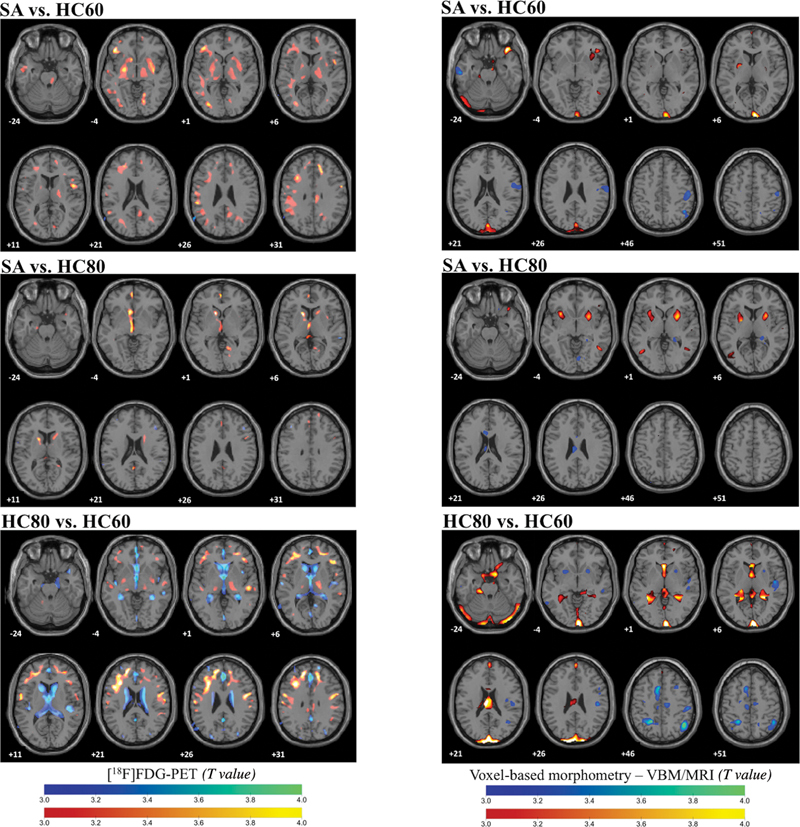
Abbreviations: GMV, gray matter volume; HC80, age-matched healthy controls; HC60, healthy controls aged 60 to 69 years; MRI, magnetic resonance imaging; rBGM, regional brain glucose metabolism; SA, SuperAgers; SPM8, Statistical Parametric Mapping 8. Note: Smaller clusters identified in the lateral ventricles and occipital cortex likely reflect partial volume effects or statistical noise. It is important to emphasize that not all presented clusters survived correction for multiple comparisons (cluster-level family-wise error correction, p
